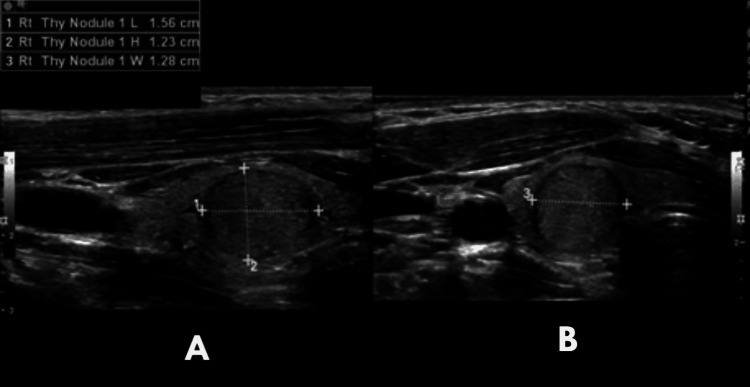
Follow-up ultrasound of the patient The image shows a slightly hypoechoic to isoechoic nodule in the right thyroid lobe seen in a follow-up study two years later. Measurement in the sagittal plane (A) was 1.56 cm x 1.23 cm. The transverse plane (B) width was 1.28 cm Rt: right. Thy: thyroid. L: length. H: height. W: width
